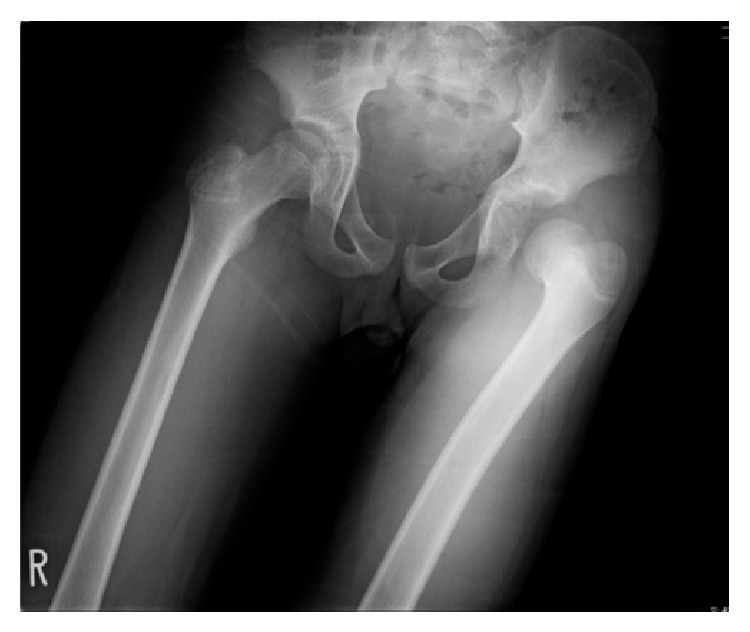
X-ray shows that the left femoral head is laterally displaced to the acetabulum, which is consistent with posterior dislocation of the hip.
